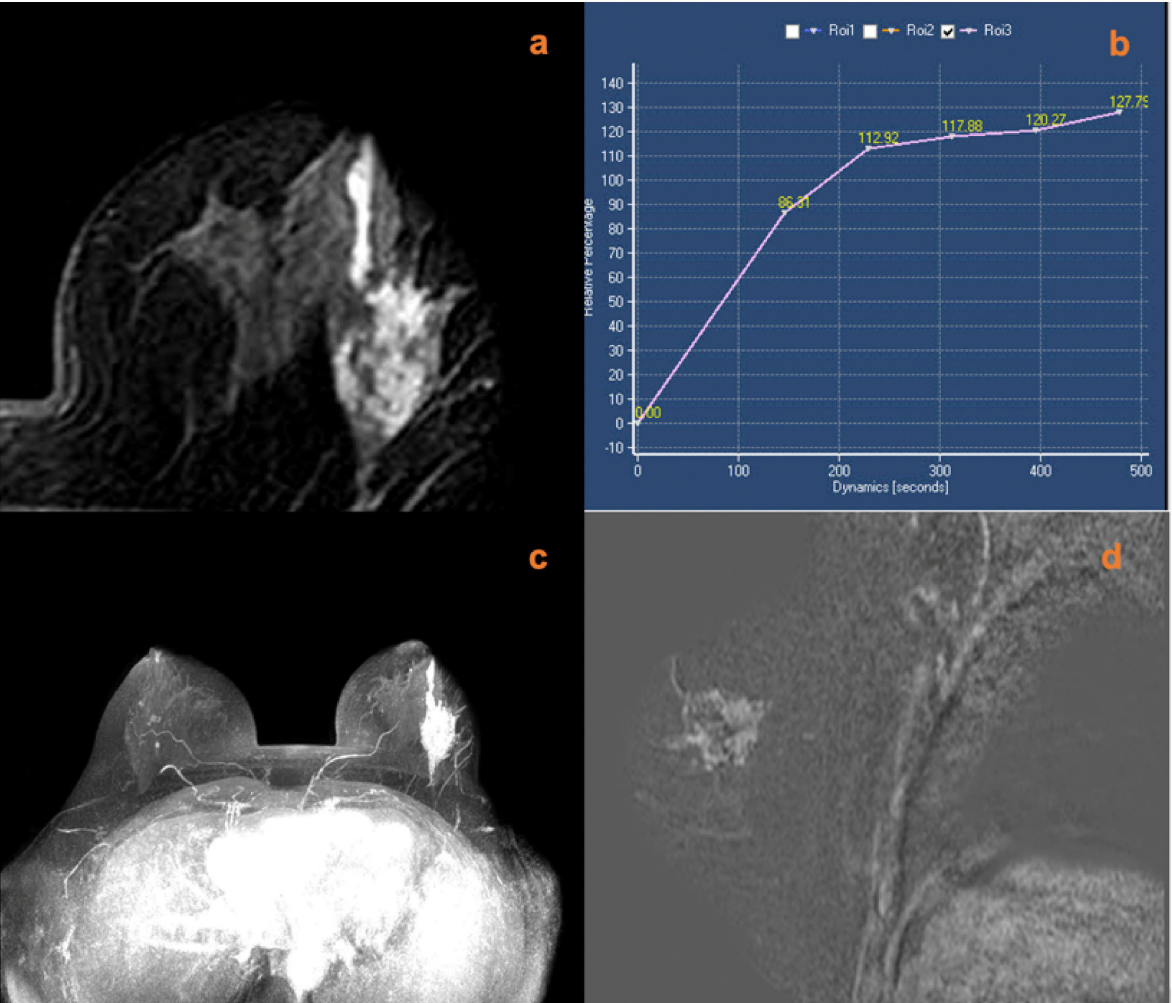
A 45-year-old female was identified with left nipple discharge for one week. MRI (A, contrast-enhanced axial; B, time signal intensity curve; C, maximum intensity projections image; D, subtraction image) shows a left breast non-mass lesion with persistent enhancement, increased ipsilateral breast vascularity, and a positive AVS sign. The KS of the lesions was 6, while the KS^*^ was 9. Postoperative pathology confirmed that the lesion was an invasive ductal carcinoma.
